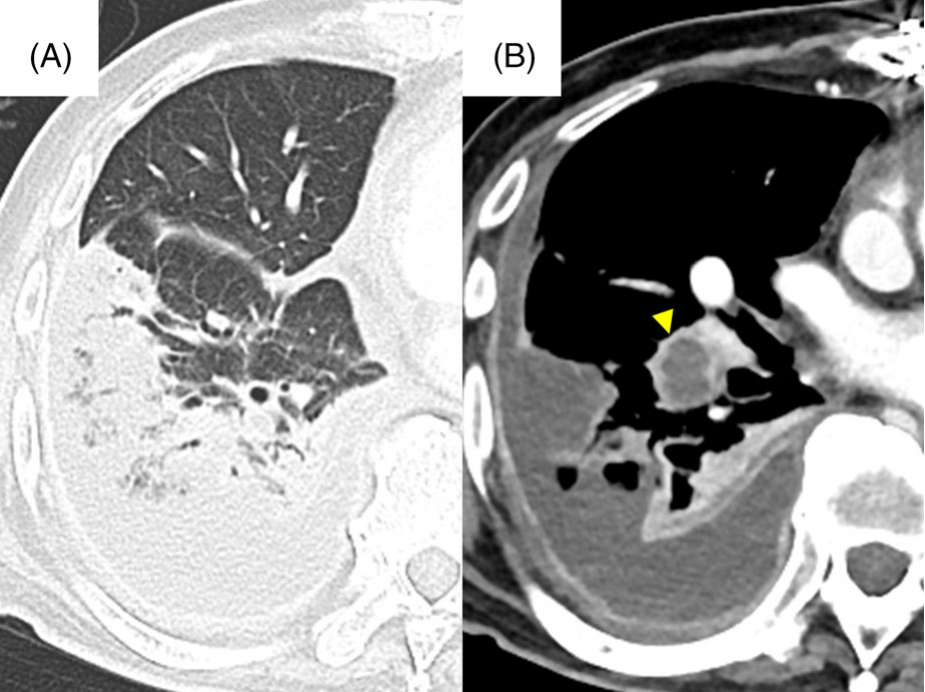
Computed tomography (CT) of the chest at 2 weeks after the embolectomy. (A) Chest CT reveals peripheral lung consolidation extending over the right lower lobe and the right pleural effusion. (B) Contrast CT shows that a contrast defect of the right basal pulmonary artery (i.e., embolus, indicated by arrowhead) remains.
